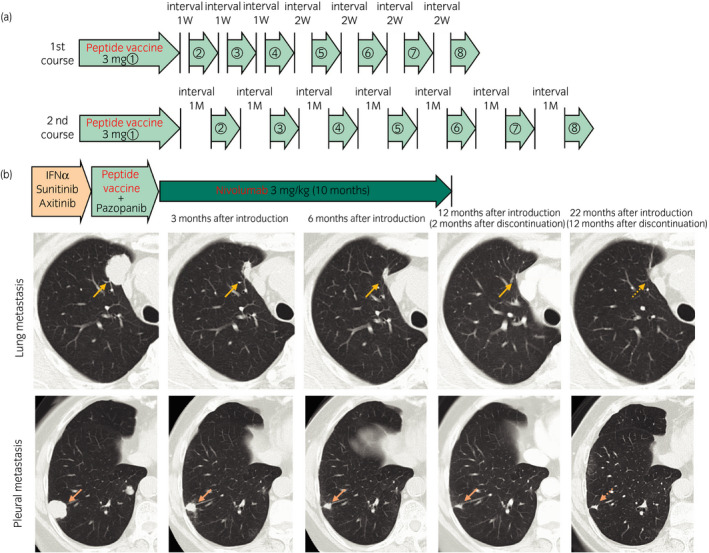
(a) Schedule of peptide vaccine administration. First course (8 doses/course): 3.0 mg/1.5 ml peptide vaccine is administrated by subcutaneous injection once per week four times, and the next four administrations are at the same dose once every 2 weeks. Second course: same dose is given once per month. (b) Chest computed tomography shows remarkable tumor shrinkage at lung and pleural metastatic sites after induction of nivolumab.
